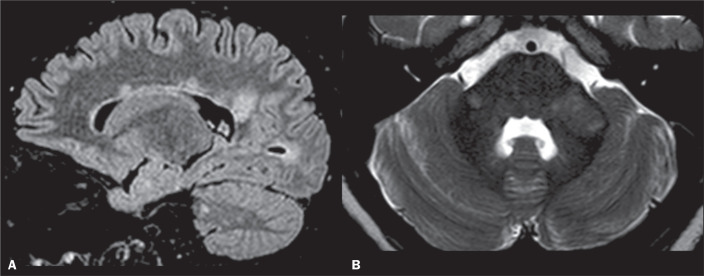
MS. A: Sagittal FLAIR sequence showing callosal lesions
